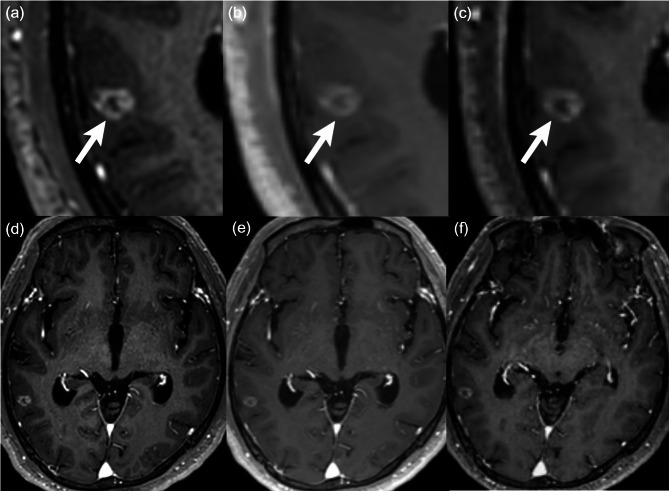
A 74-year-old man with lung cancer. As compared with the baseline image (a), a small contrast-enhancing lesion (arrow) at the right temporal lobe appears slightly less discrete due to a lower degree of lesion-to-gray matter contrast on the original FU image (b). On the harmonized FU image (c), the lesion appears similar to that of the baseline image in terms of the border and contrast enhancement. Note that the gray matter-to-white matter contrast is lower on the original FU image (e) than on the baseline (d) or harmonized FU image (f). The baseline and FU images were scanned with Ingenia 3.0T CX (Philips Healthcare) and Magnetom Skyra (Siemens Healthineers) MRI scanners, respectively.
